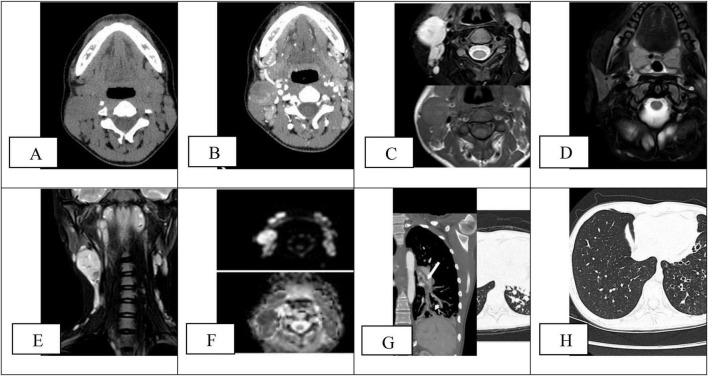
(A,B) Multiple enlarged lymph nodes in bilateral cervical regions, predominantly on the right side. The lymph node shows abnormal morphology, are globularly enlarged, and demonstrate heterogeneous enhancement. The surrounding fat planes are well-defined, with no obvious exudation. (C–F) MRI T2-weighted images demonstrated focal nodular mucosal thickening in the right pharyngeal recess, with restricted diffusion on DWI. Bilateral cervical lymphadenopathy, predominantly right-sided, showed heterogeneous T2 signal without infiltration of surrounding fat. (G,H) Showed: multiple bronchodilatation, with mucusclots (short arrow) and bronchial artery dilation (long arrow). (G, March 16, 2024); Re-examination after treatment with Sirolimus. The effect is remarkable (H, January 25, 2026).
